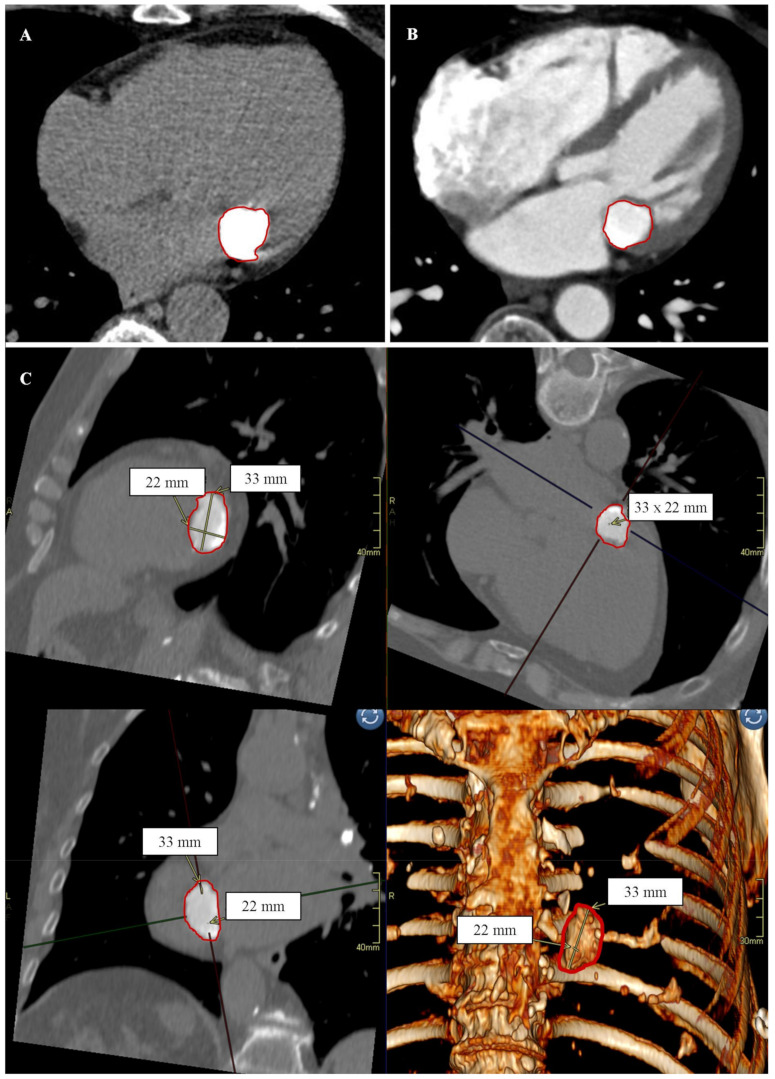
Patient No. 1. Caseous calcification of the mitral annulus (CCMA) in cardiac computed tomography. (A) Native phase. Axial reconstruction. The borders of the CCMA are marked in red. (B) Post-contrast phase. Axial reconstruction. The borders of the CCMA are marked in red. (C) Post-contrast phase. Secondary reconstructions. From the top and left side sequentially: MPR reconstruction, view in the short axis; MPR reconstruction, view in the long axis, 4-chamber; MPR reconstruction, view in the long axis, 2-chamber; VRT reconstruction. CCMA dimensions are marked with yellow lines and arrows; CCMA borders are marked in red.
